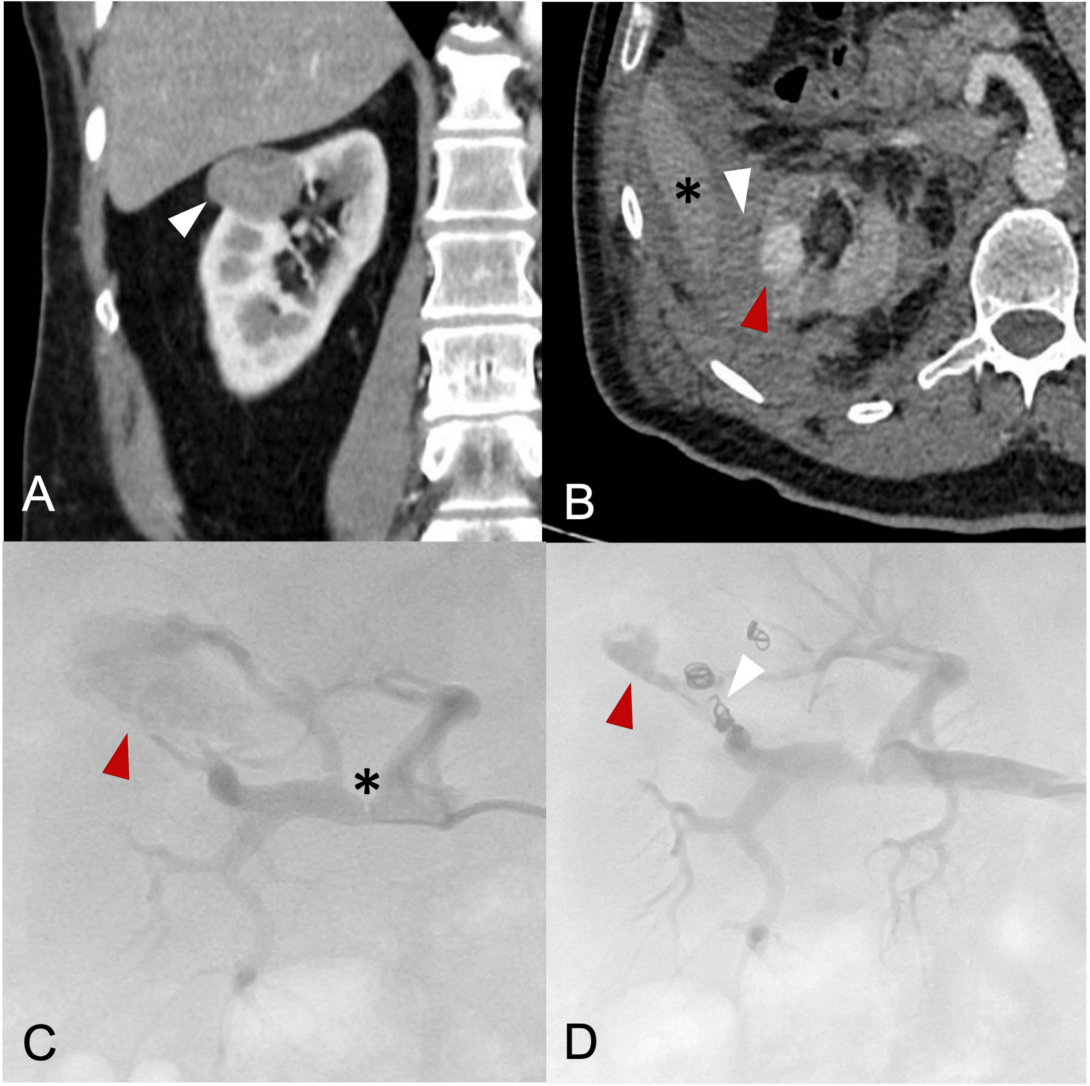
CT and Angiography A: Coronal CT, neoplastic lesion in the right kidney (arrowhead). B: Axial CT, pseudoaneurysm (red arrowhead), hemorrhage in Morrison's pouch (white arrowhead), liver (asterisk). C: Pre-embolization angiography, AP projection of right kidney, pseudoaneurysm (arrowhead), stenosis (asterisk). D: Post-embolization angiography, AP projection of right kidney, small remnant (red arrowhead), and microcoils (white arrowhead). AP, anteroposterior.
